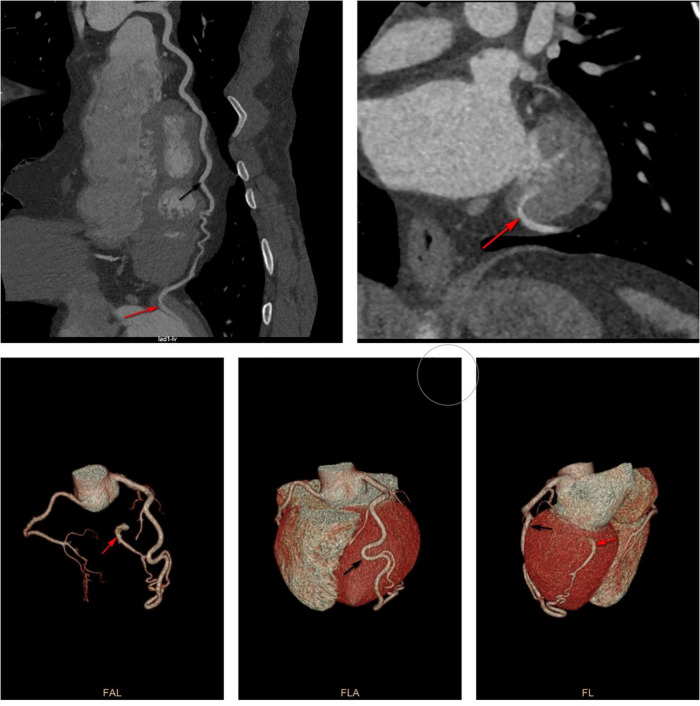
Computed tomography angiography showed that the diagonal branch of the left coronary artery was twisted, lengthened, expanded, extended along the left heart margin, and its distal end penetrated the myocardium from the basal segment of the left ventricular posterior edge into the left ventricle. Black arrow shows the thick diagonal branch; red arrow shows coronary artery-left ventricular fistula.
